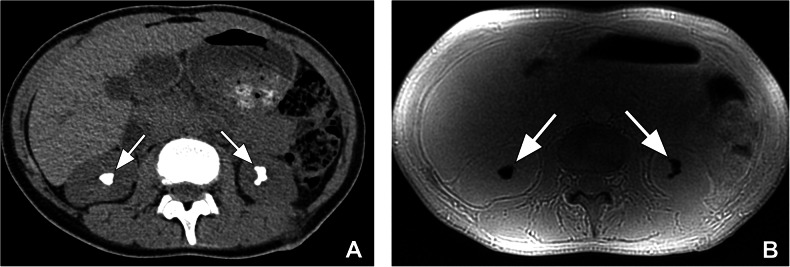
A 15-year-old girl with bilateral kidney stones. A Axial non-enhanced abdominal CT image shows lower pole stones in the right and left kidneys (arrows). B Axial ZTE-MR image at the same level demonstrates kidney stones (arrows)
